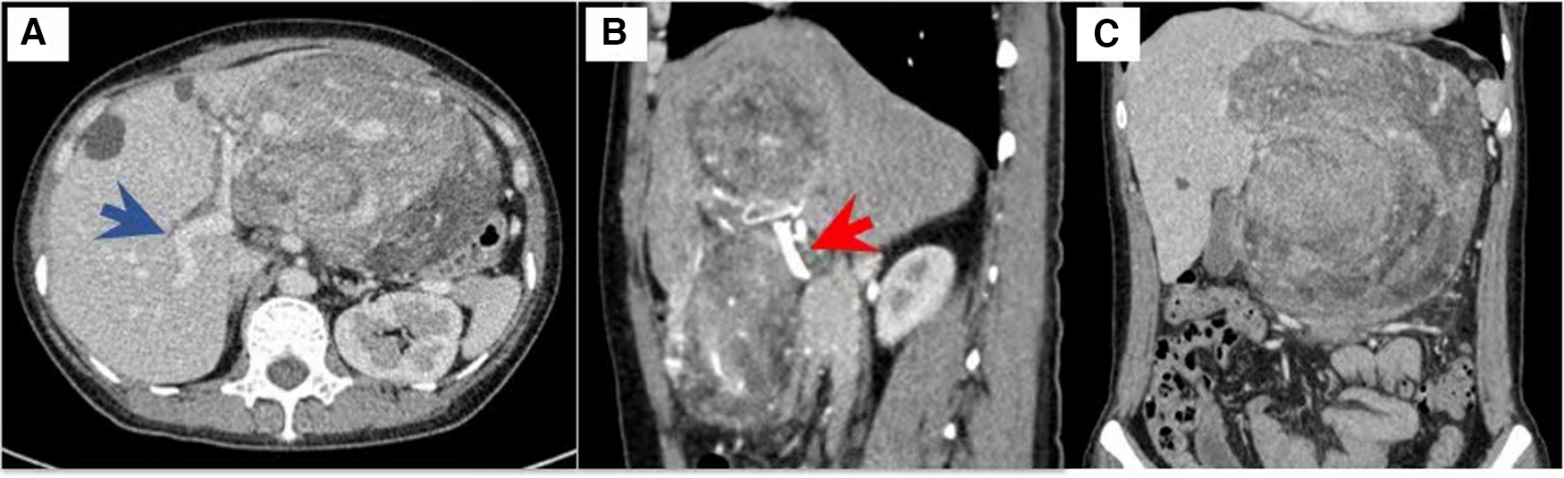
Contrast-enhanced computed tomography of the mass. Blue arrow, portal vein. Red arrow, hepatic artery.
